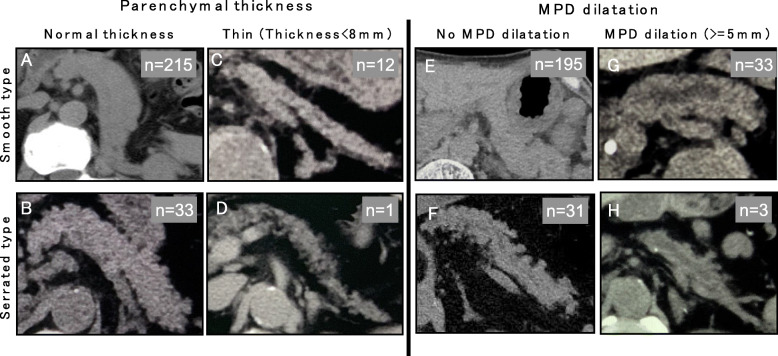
Morphology and contour of pancreas preoperative plain CT. We divided the pancreatic CT configuration into a smooth type (Upper lane) and serrated type (lower lane). a. Smooth type in the pancreas with normal thickness. b. Serrated type in the pancreas with normal thickness. c. Smooth type in the thin pancreas. d. Serrated type in the thin pancreas. e. Smooth type without dilatation of MPD. f. Serrated type without dilation of MPD. g. Smooth type with dilation of MPD H. Serrated type with dilation of MPD MPD: main pancreatic duct
